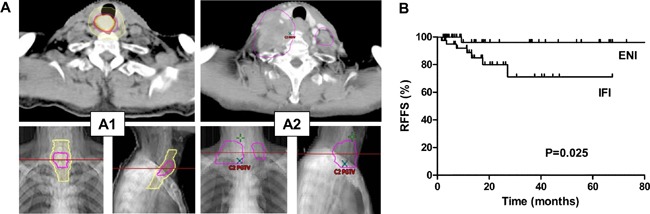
Local-regional nodal failure after radiotherapy and the omission of prophylactic nodal coverage (A) Example of supraclavicular LN metastasis cases after treatment. A1: Primary tumor (highlight orange) identified by positron emission tomography-computed tomography (PET-CT), including both gross tumor volume (GTV, magenta) and clinical target volume (CTV, yellow); A2: The occurrence of supraclavicular LN metastasis after treatment (fuchsia). (B) Relationship of regional failure-free survival (RFFS) and CTVn delineation method. ENI, elective nodal irradiation, IFI, involved field irradiation.
